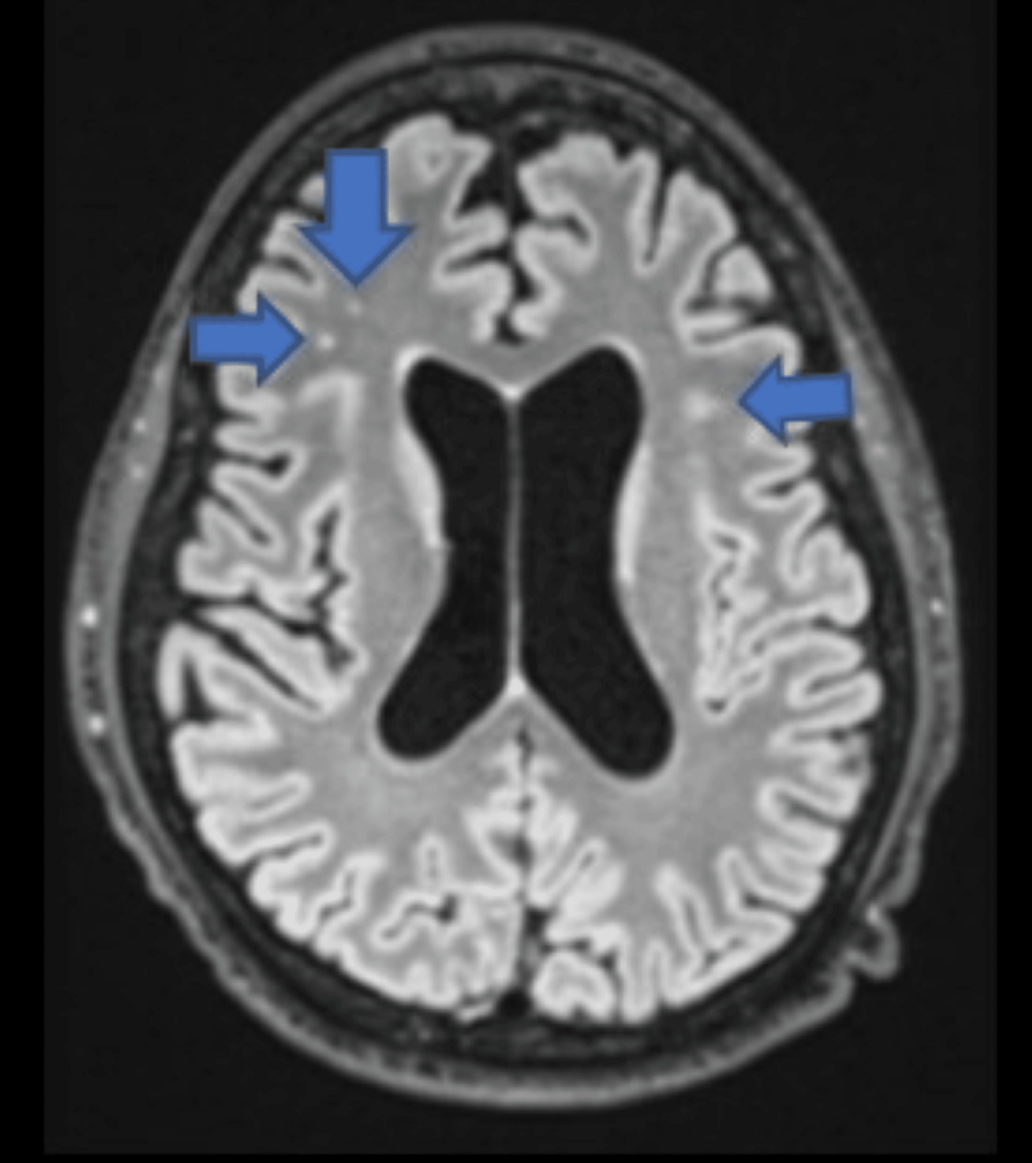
MRI of the brain three weeks post-arrest MRI T2/FLAIR of the brain revealing punctate foci of T2 hyperintensity within the subcortical white matter.
